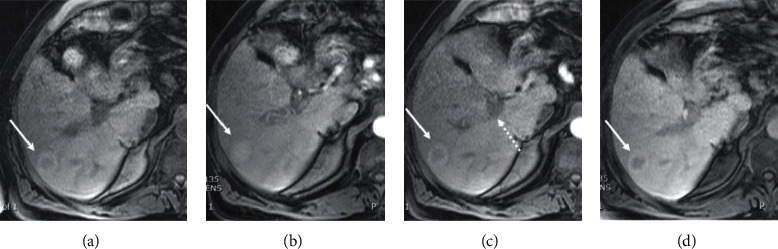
MRI with Eovist® (gadoxetate disodium contrast) shows the persistent portal vein thrombosis (dotted arrrow) and the segment 7 lesion (solid arrow). The lesion measures 2.6 cm and is faintly hypointense with hyperintense rim on precontrast T1W image (a), enhances centrally on arterial phase (b), central washout with a persistent hyperintense rim on portal venous phase (c), and no Eovist® uptake centrally with a persistent hyperintense rim on hepatocyte phase. This appearance is suspicious for HCC.
